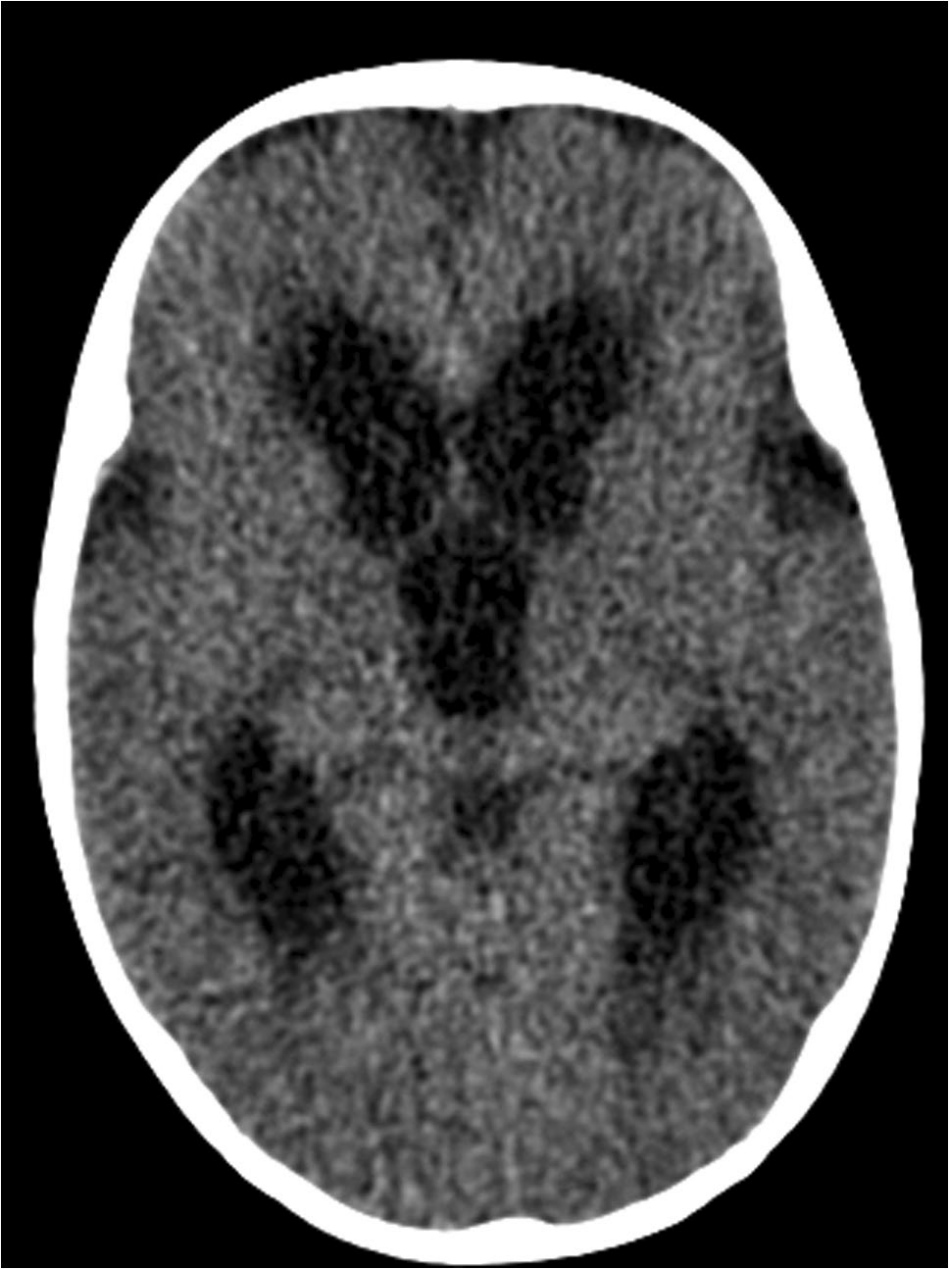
Axial non-contrast computed tomography scan of the brain in a 2-year-old boy with severe hydrocephalus due to tuberculous meningitis
